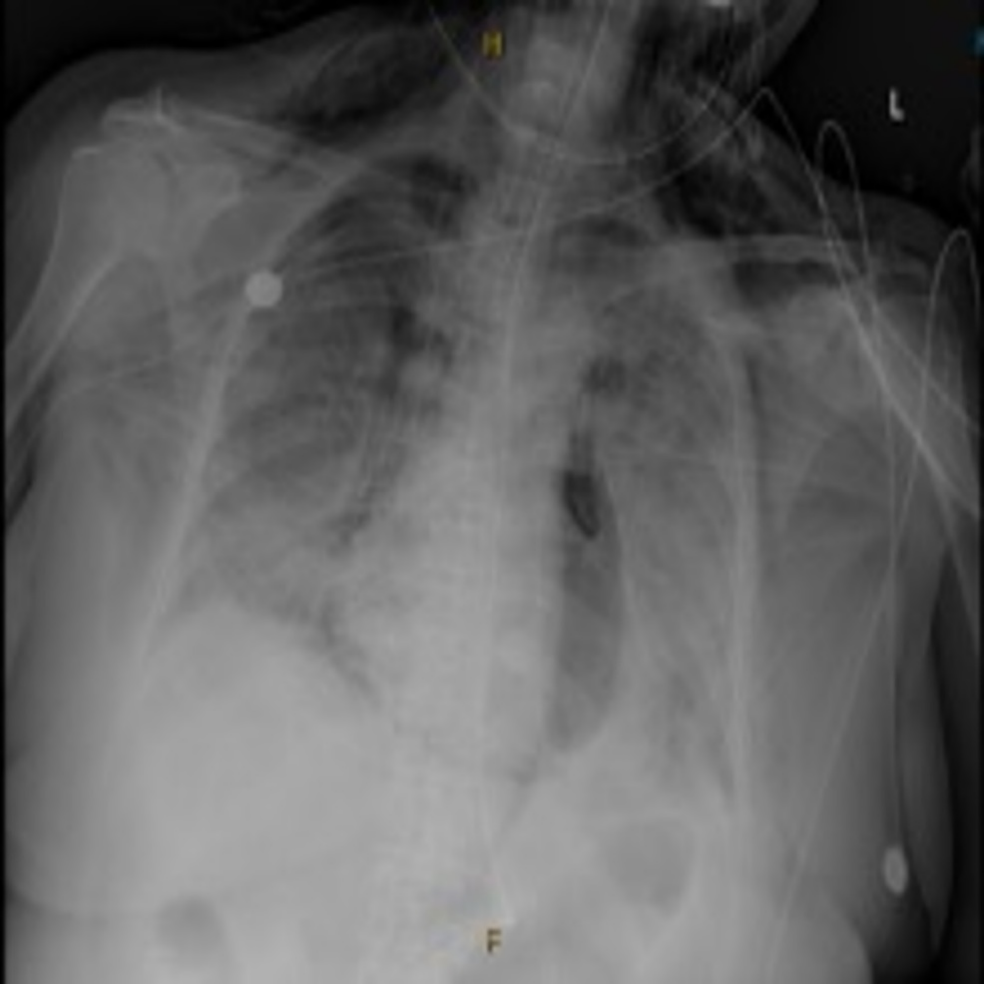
Post intubation chest X-ray. Post intubation chest X-ray showing both pneumothorax of the right lung and pneumopericardium.
